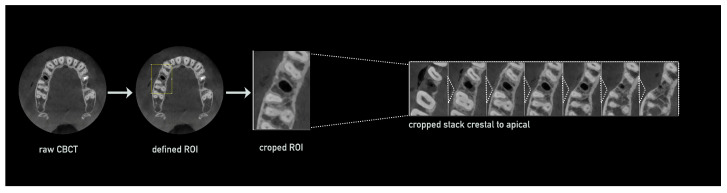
Semi-automated CBCT processing in preparation for bone width evaluation. The raw CBCT data were imported, followed by the definition of the region of interest (ROI) and cropping to isolate the area including the treated socket. The resulting stack encompassed the entire region from the crestal to the apical aspect.
